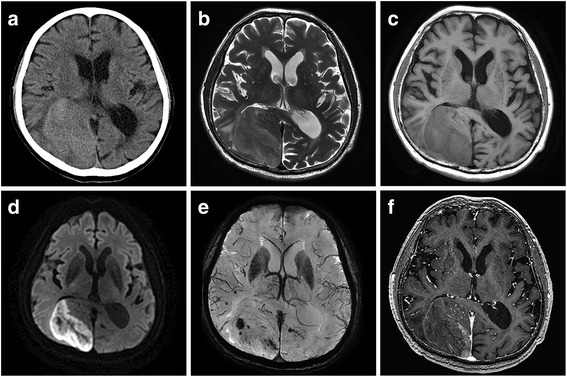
Axial brain CT and MRI. CT shows the extra-axial mass in the left posterior lobes (a). MRI shows hyperintensity on T2WI (b), hypointensity on T1WI (c), marginal hyperintensity on the diffusion-weighted image (d), intra-tumor hemosiderin deposits on T2*WI (e), and heterogeneous hyperintensity on gadolinium-enhanced T1WI (f)
